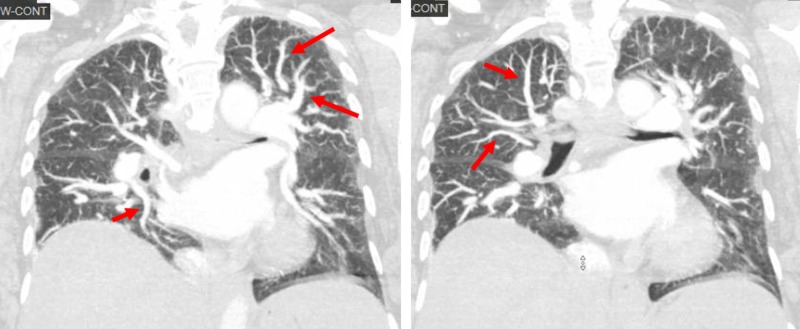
Computed tomography of the chest with intravenous contrast. Prominent pulmonary vascular dilatation extending from hilum to the periphery of the lung.
